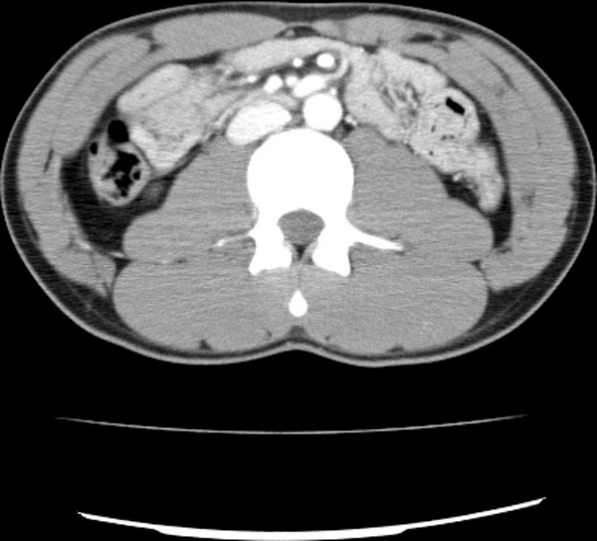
Axial CT scan obtained with IV contrast material in 17-year-old male control subject.
